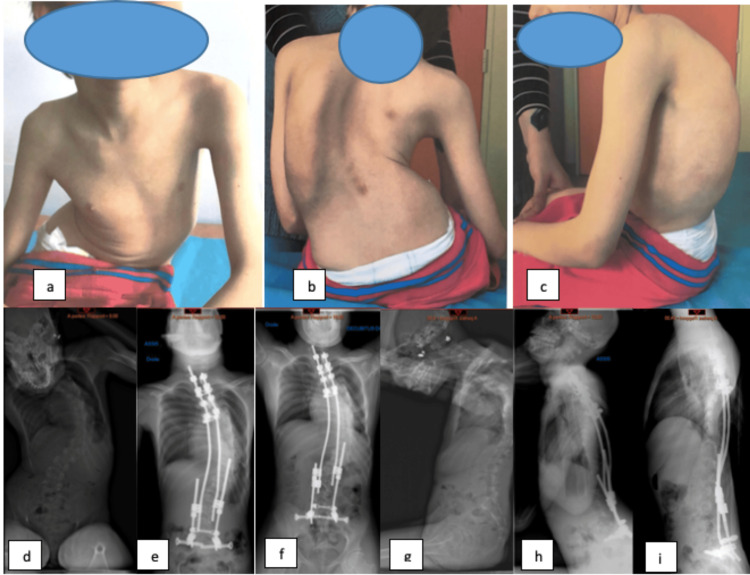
Clinical pictures Example of a patient with cerebral palsy: preoperative clinical aspect from the front (a), back (b), and side (c); radiographs from the front preoperatively (d), immediately postoperatively (e), and four years later (f); and from the side preoperatively (g), immediately postoperatively (h), and four years later (i)
